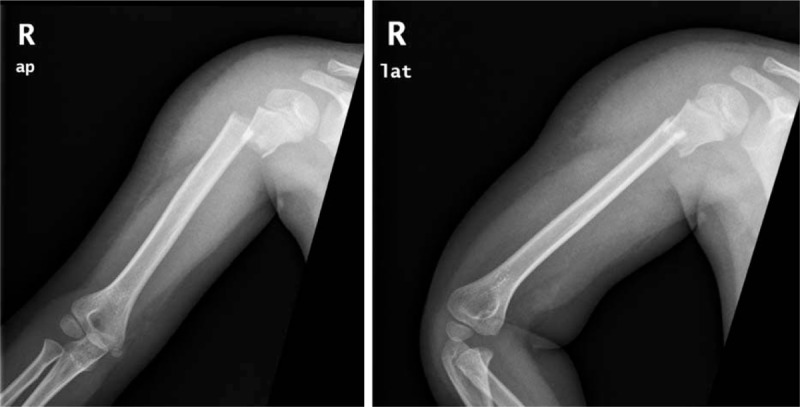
Preoperative anteroposterior and lateral x-ray showing displaced metaphyseal fracture of the right proximal humerus without the sign of shoulder dislocation.
